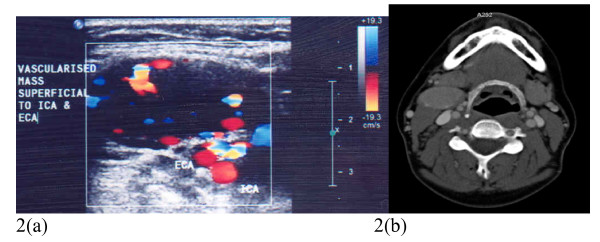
2A: Carotid duplex image showing a highly vascular mass superficial to the ICA and ECA. 2B: Axial CT of the neck post IV contrast showing a well circumscribed enhancing homogenous mass in the right parapharyngeal space.
